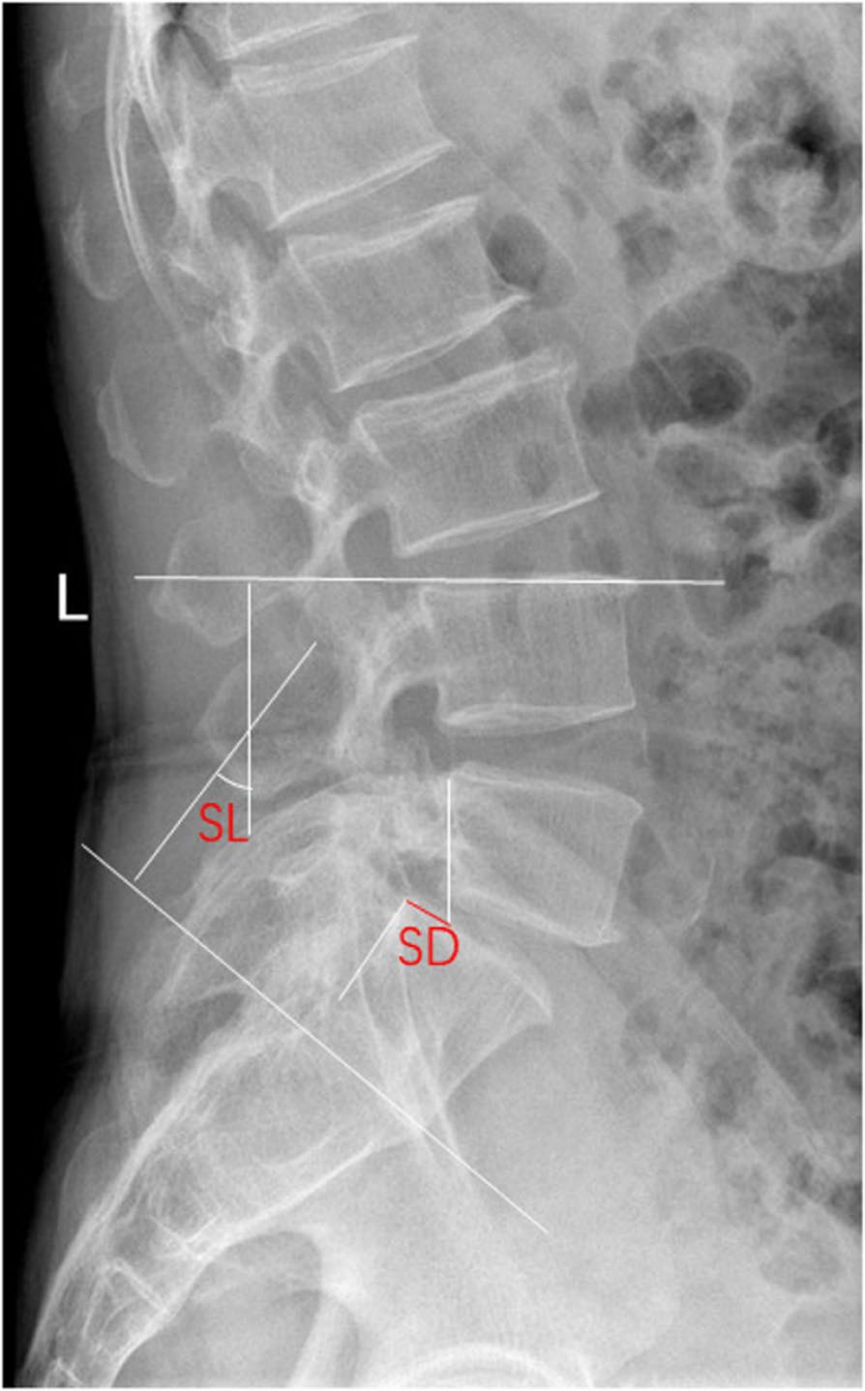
Plain lateral radiographs for measuring local parameters. SD: Slip degree, SL: Segment lordosis
